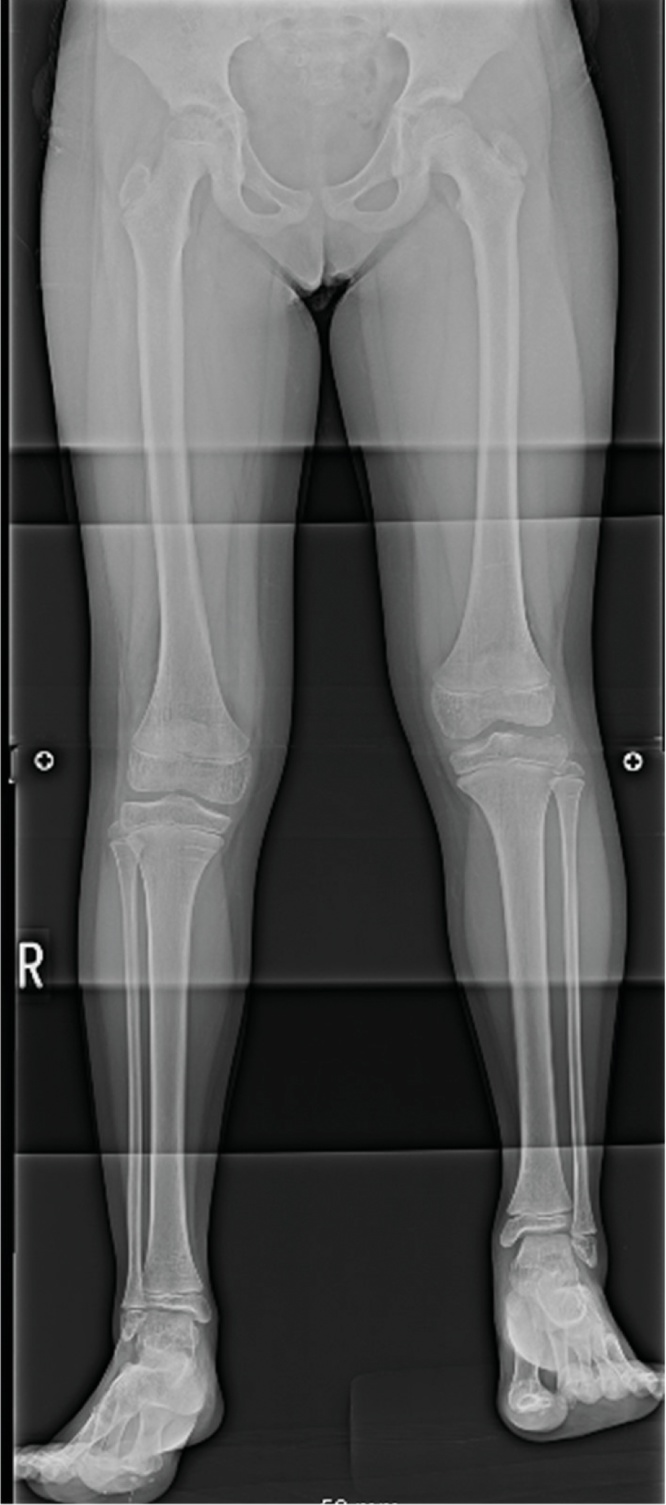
Plain radiographs of both lower extremities. Plain radiography showed that limb inequality remained even 1 year after tumor resection.
